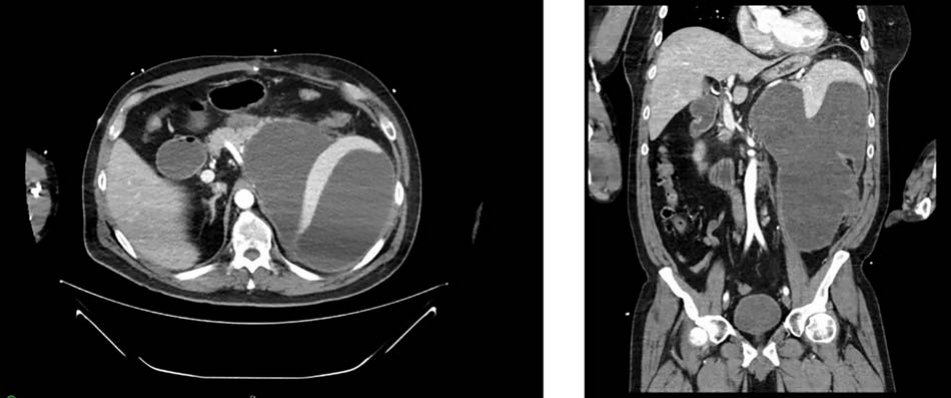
Appearance of effusion in the left retroperitoneal space in transverse (left) and coronal (right) planes on CT; it can be seen encroaching on the spleen; subsequent cytology ruled of malignant effusion.
